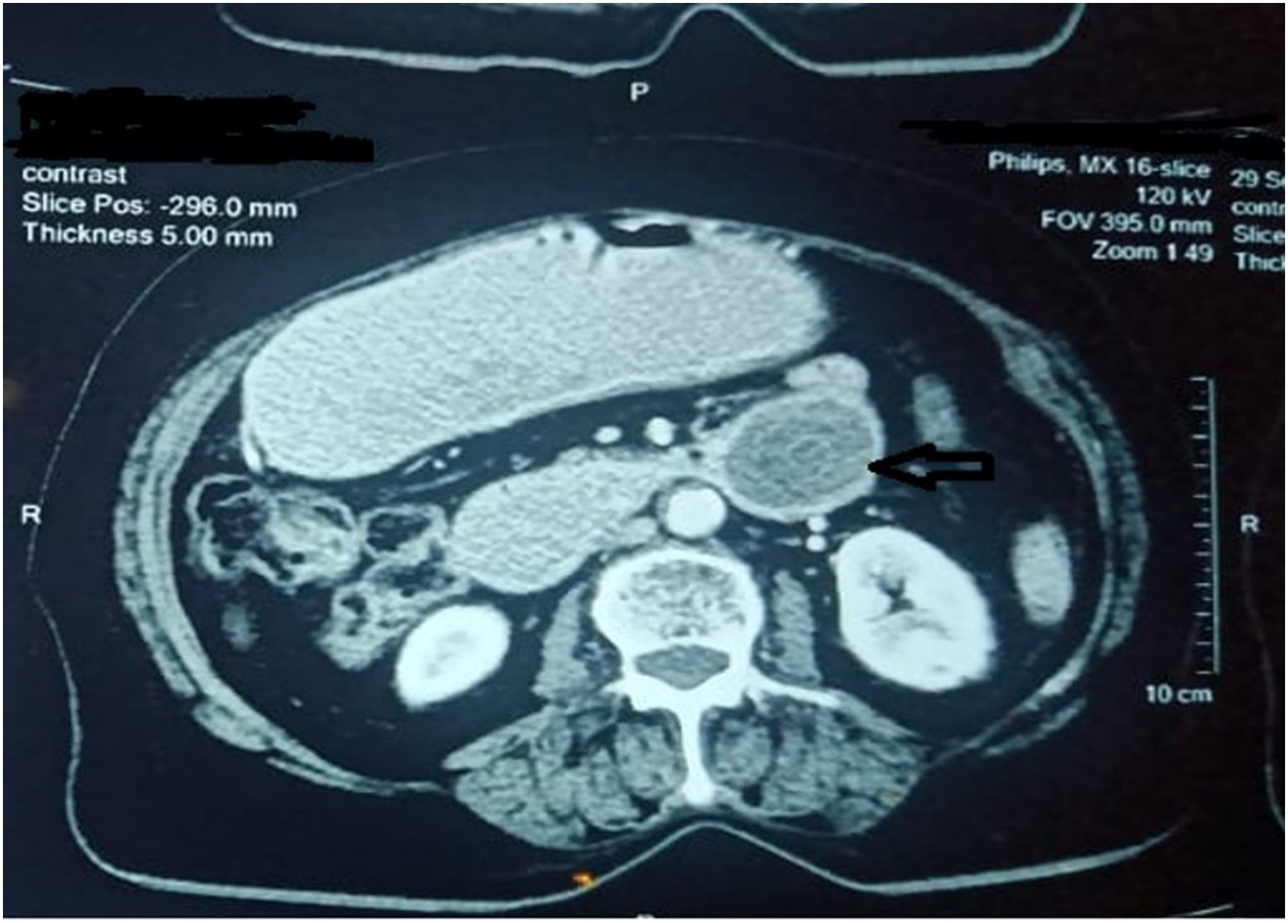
Contrast‐enhanced CT showing a large stone in a whorled appearance at the duodenojejunal junction (black arrow).
